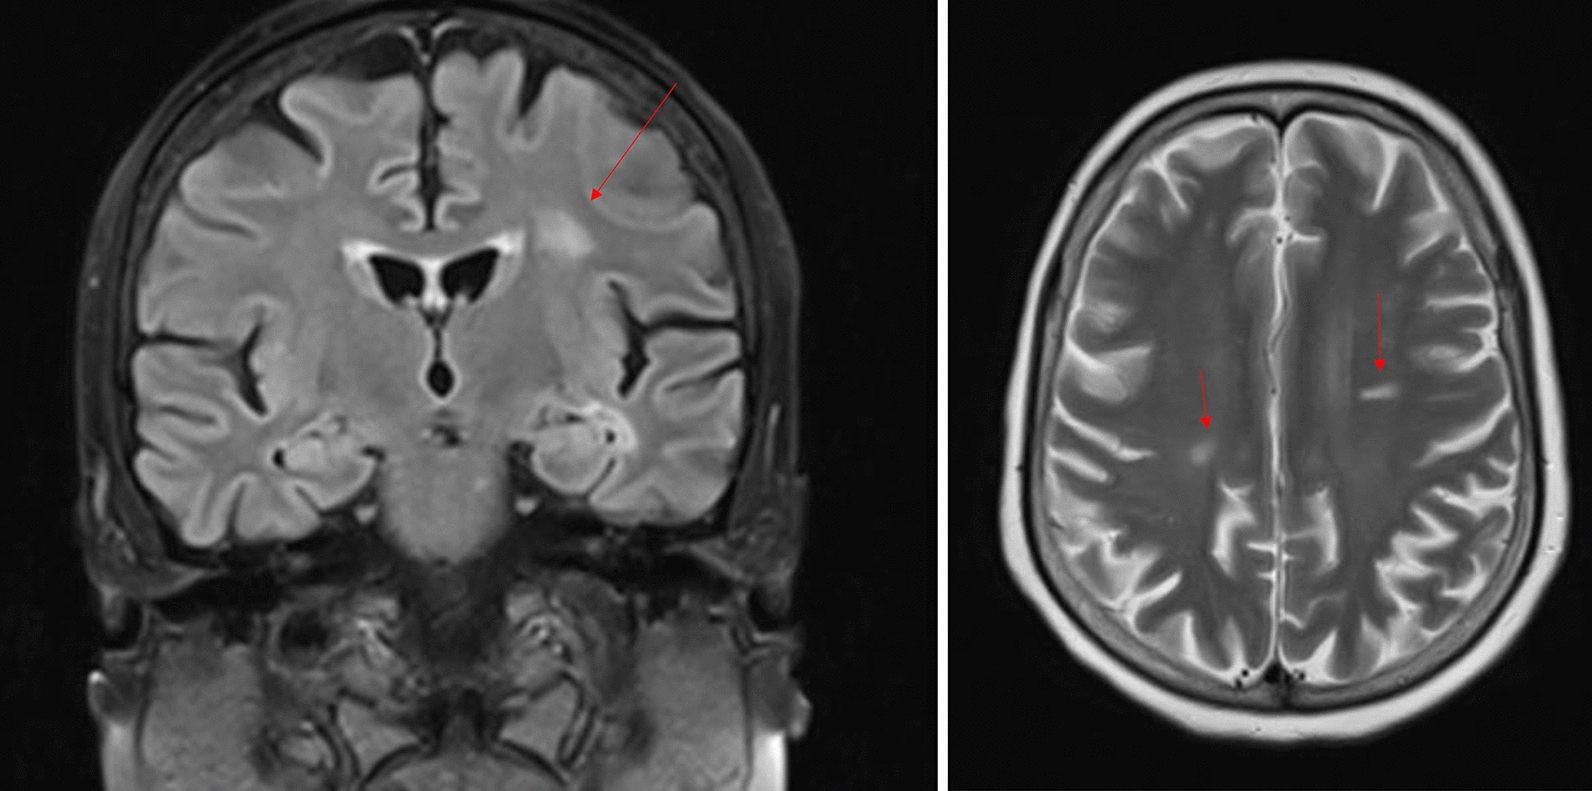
Brain MRI showing demyelinated subcortical plaques consistent with multiple sclerosis. Coronal (left) and axial (right) FLAIR-weighted images demonstrate multiple hyperintense lesions (red arrows) within the periventricular and subcortical white matter, characteristic of demyelinating plaques. No evidence of intracranial metastasis was detected
